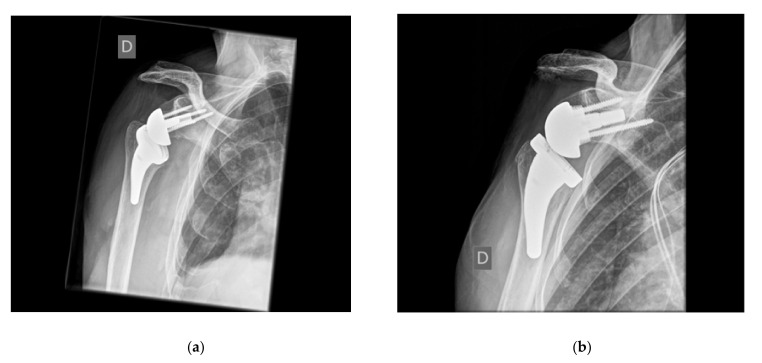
AP radiographs with increased glenoid-sided offset: (a) Bony, (b) Metal. Minor valgus implantation of stems.
